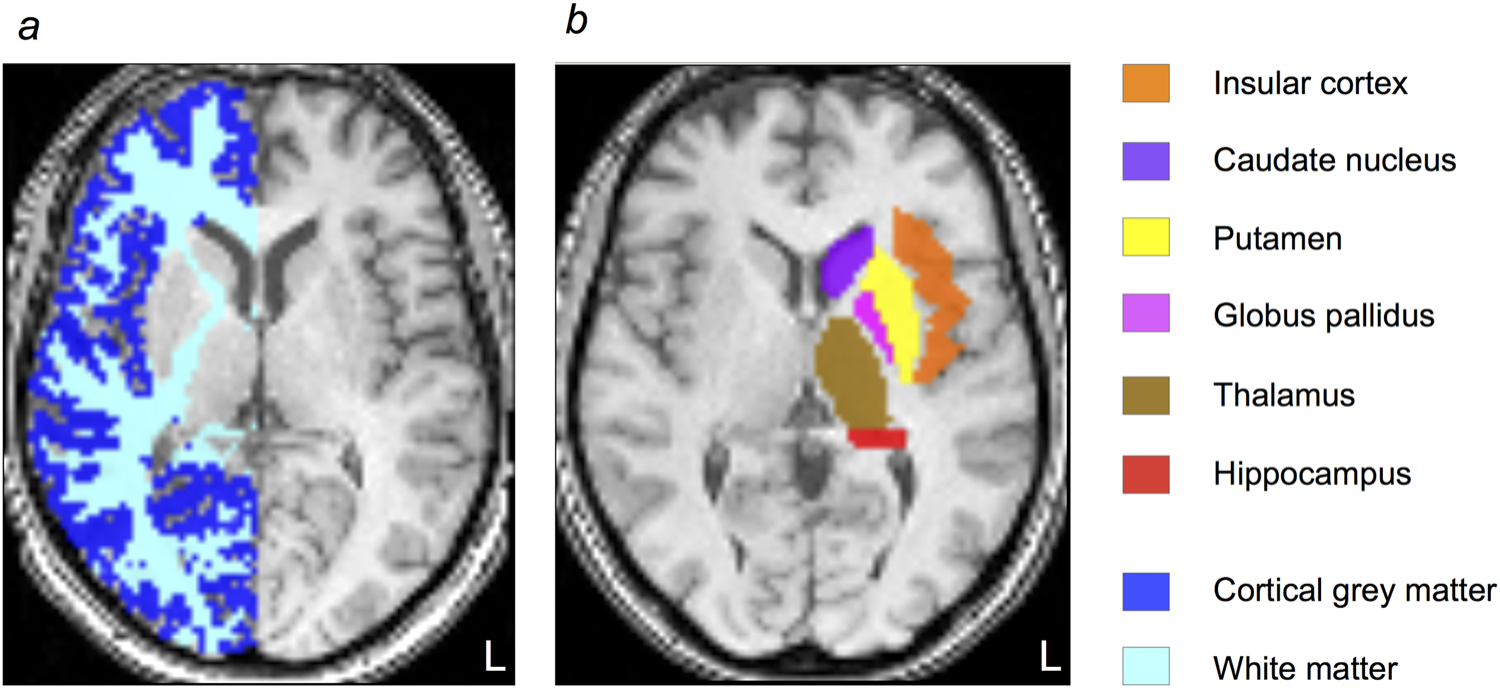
Analyzed volumes of interest. (a) Automatically segmented individual grey and white matter VOIs on a spatially normalized axial T1-weighted image of a subject with left hemispheric tumor (male, 39 years old). (b) Anatomical volumes of interest (VOIs) on a spatially normalized axial T1-weighted image of a subject with right hemispheric tumor (female, 39 years old). For each subject, only the hemisphere contralateral to the lesion was analyzed, generating mean values for a total of 8 VOIs per subject.
